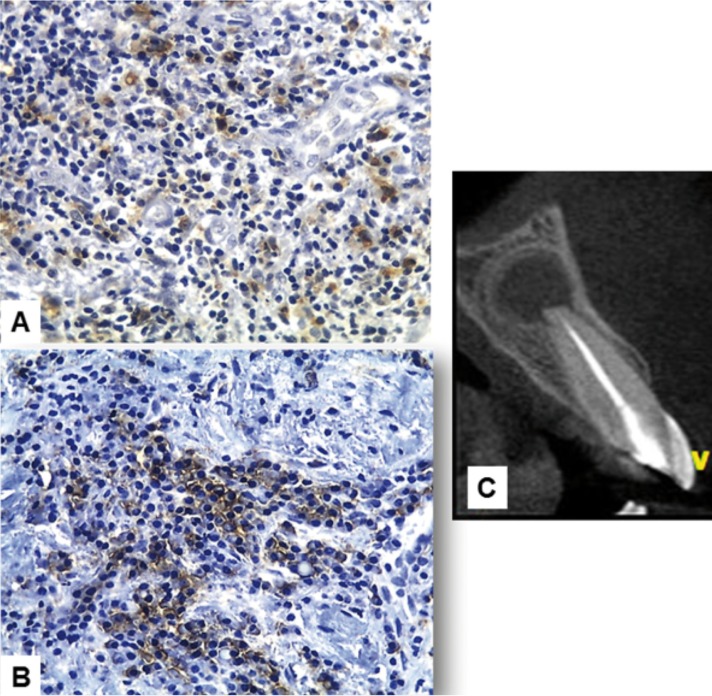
Periapical cyst. A: CD68+ macrophages. B: CD138+ plasma cells (immunoperoxidase, original magnification, x400). C: tomographic image.
